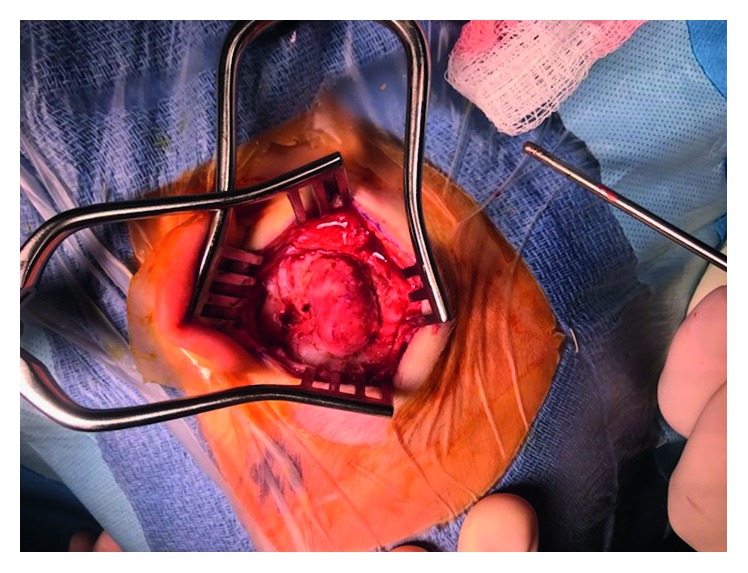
In vivo appearance of MNTI, demonstrating significant progression from 1.3 cm nodule on T1-contrasted sequence MRI two weeks prior. Note that a cortical mastoidectomy has been performed at the anterior (left) aspect to allow identification of the mastoid tegmen and landmarks for resection.
